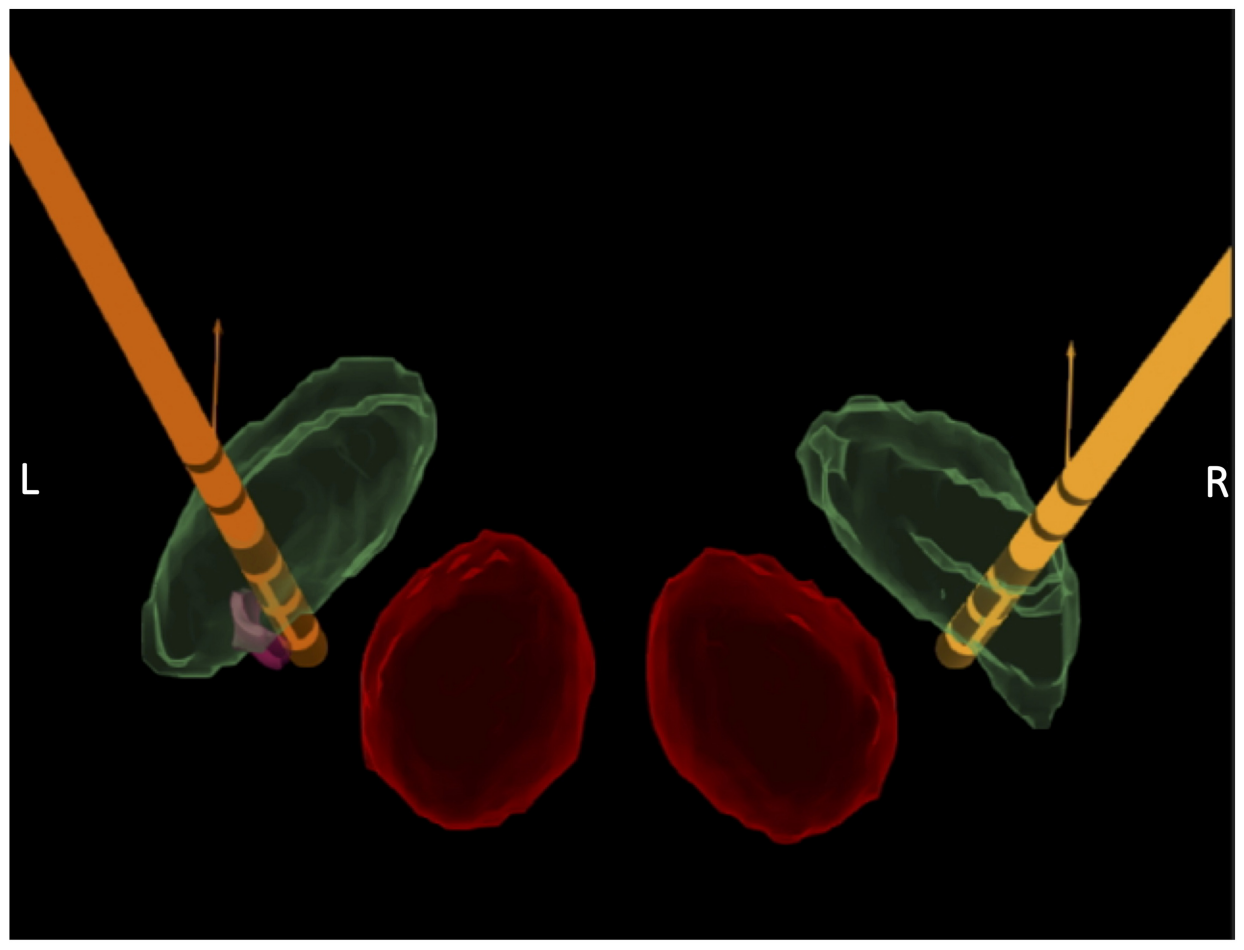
Lead location confirmation. Fusion of postoperative CT scan with preoperative 3T axial MRI, using Elements^©^ software (Brainlab^©^) and Guide XT^©^ software (Boston Scientific, Valencia, California), showing the final position of bilateral directional leads (orange) within the subthalamic nucleus (STN; green) and the red nucleus (red). The left lead was slightly medialized inside the STN and a small volume of tissue activated (VTA; pink sphere) was generated close to contact 3, to highlight its position in the ventromedial region of the nucleus.
